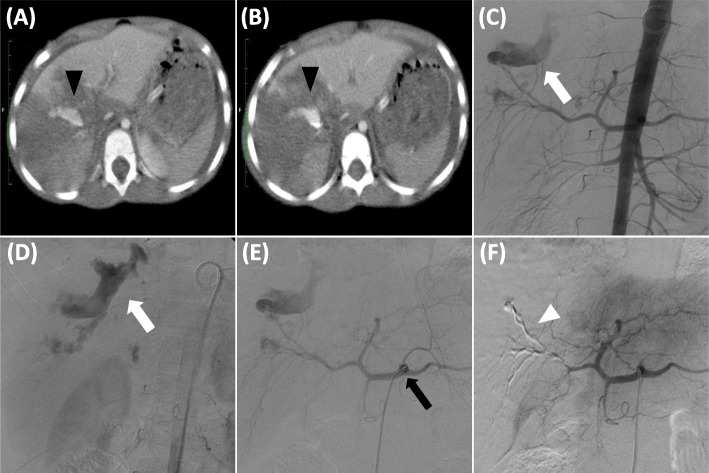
(a, b) CT image arterial phase after endovenous contrast injection, depicting contrast deposition inside the right liver lobe (black arrowhead). (c, d) DSA with Pigtail cateter injection in aorta showing contrast extravasation (white arrow), seen in image (e) with a DSA performed by a Cobra 2 catheter (black arrow) in the celiac trunk. (f) After embolization, glue cast (white arrowhead) in a subtracted image in the liver territory after embolization with complete superseletiva exclusion of the injured vessel from the circulation
